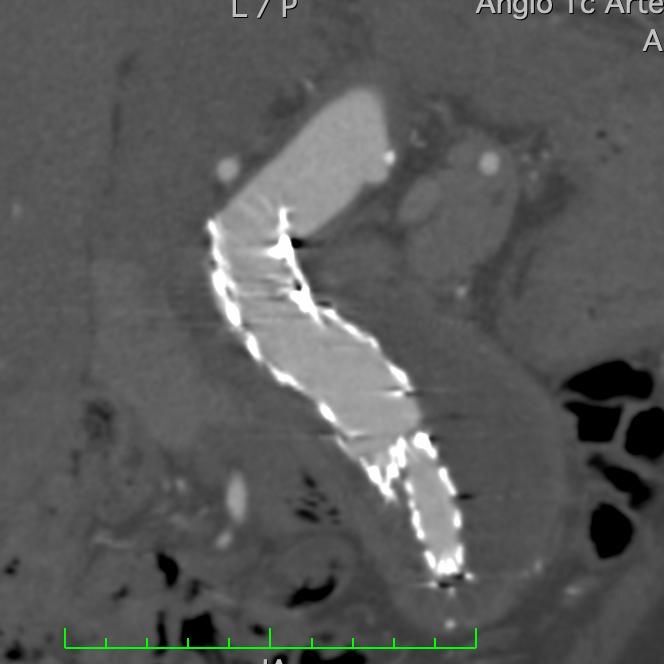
CT angiography image 30 days after EVAR with the Neoneck technique, showing rectification of the aortic neck, with no endoleaks.
